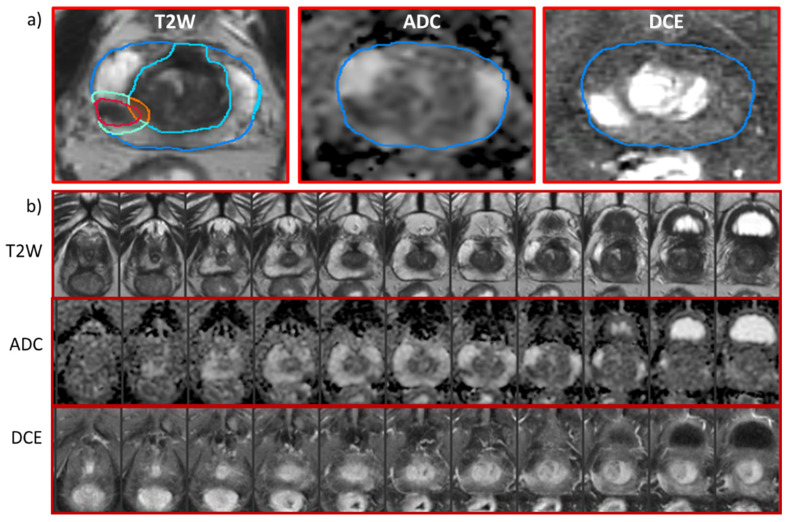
MpMRI sequences and segmented ROIs for analysis. (a) Representative axial slices from mpMRI of a patient at a baseline: T2-weighted, ADC, and early enhancing DCE-MRI series. The contours in the T2-weighted image are of the prostate (blue) and PZ (cyan). Gross Tumor Volume (GTV) is outlined in red; peri-tumoral GTV is represented by turquoise within the peripheral zone (PT-PZ) and orange contour within transitional zone (PT-TZ). (b) Axial slices through the entire prostate from apex to base on T2-weighted, ADC and early enhancing DCE-MRI series. The eight slice (from left to right) corresponds to the image in panel a).
